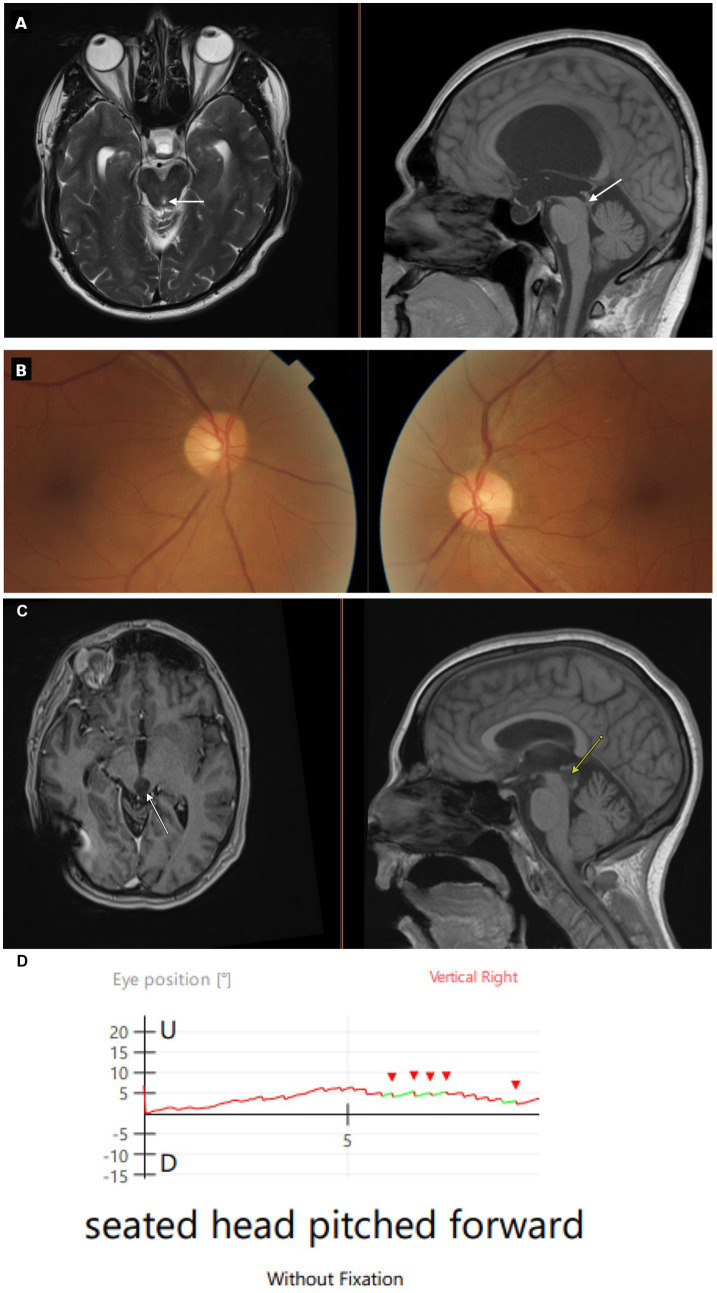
Clinical data. (A) Case 1 – MRI (axial T2-weighted left, sagittal T1-weighted right) demonstrates marked third and lateral ventricular dilatation, normal fourth ventricle caliber, and distal cerebral aqueduct narrowing (arrows). (B) Case 1 – Fundus photographs showing intorsion of the left eye (right side of photograph) and extorsion of the right eye (left side of photograph). (C) Case 2 – MRI (axial T1-weighted left, sagittal T1-weighted right) shows a non-enhancing tectal lesion involving the quadrigeminal plate to the left of the midline, with cerebral aqueduct compression. (D) Case 2 – Vertical eye position during head pitch forward (no fixation). Right-eye trace shows upward slow-phase drift with corrective downward quick phases (red carets).
